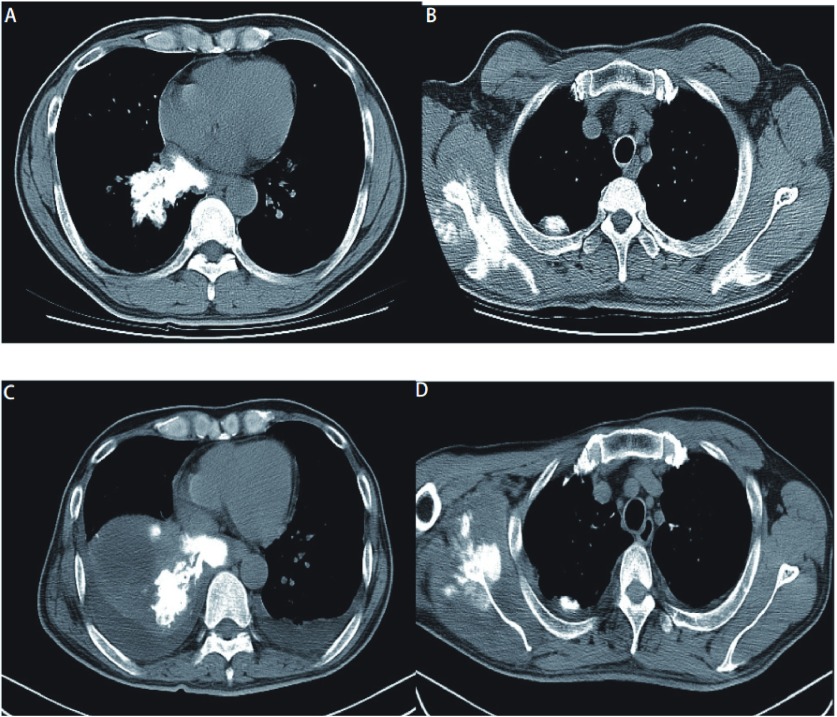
患者治疗前后胸部CT。A：右肺下叶一不规则肿块影，其内清晰支气管影，管壁钙化；B：右肩胛骨可见骨质破坏，周围软组织见多发斑片状高密度影；C、D：治疗后，右肺下叶肿块影较前变大，并出现双侧胸腔积液 The chest computed tomography (CT) before chemotherapy and after chemotherapy. A: CT showing an irregular tumor in the inferior right lobe, in which we can see the clear bronchitis shadow and calcification of tubal wall; B: CT showing the destruction of the right scapula and patching high density shadow in surrounding soft tissue; C, D: after the chemotherapy, showing an enlarging tumor in the in ferior right lobe and bilateral pleural effusion
